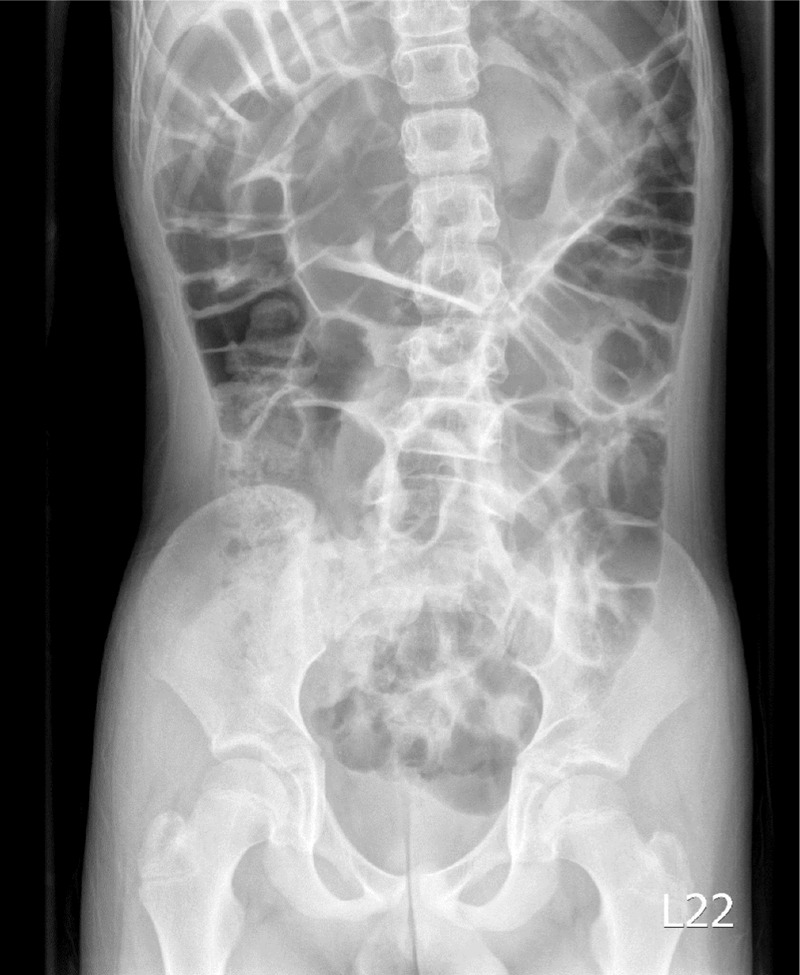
Radiograph demonstrating a greatly dilated sigmoid that almost filled the entire abdomen 1 day before admission.
